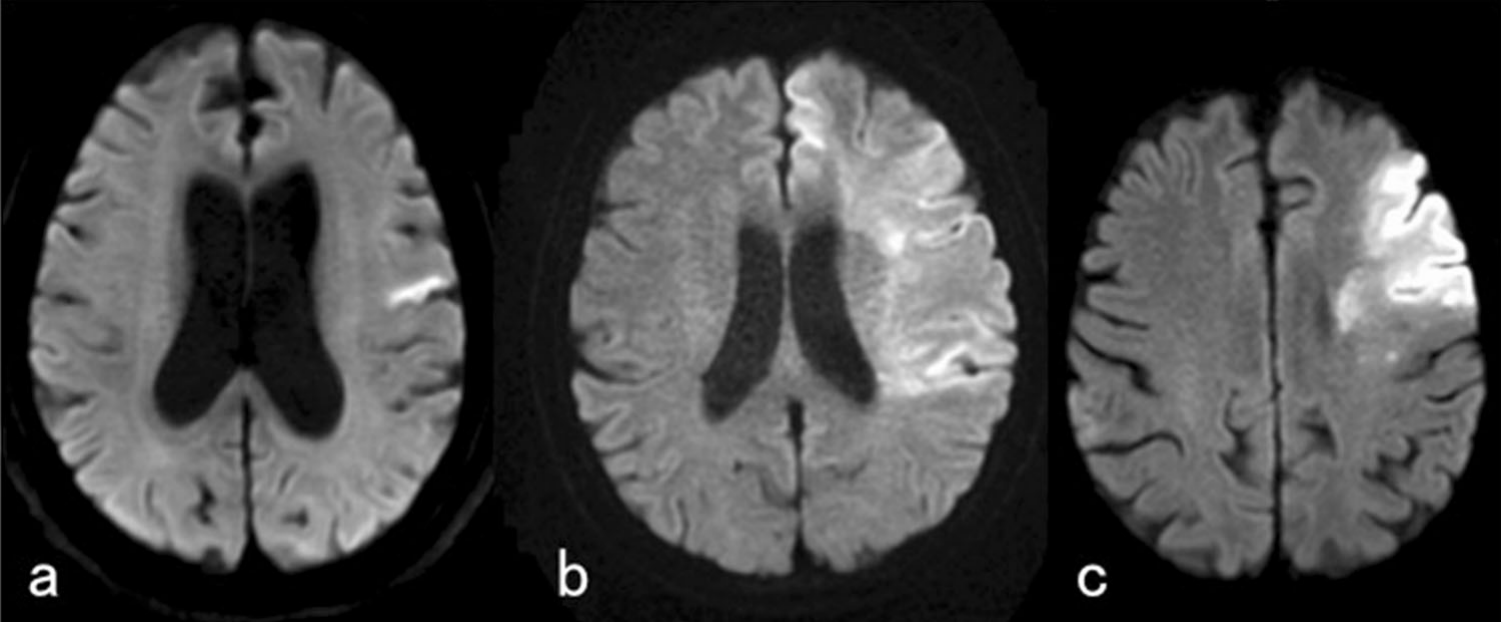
Diffusion-weighted magnetic resonance imaging (DWI) of three representative cases. a Case 1 demonstrated a left hemisphere lesion including the entire frontal lobe, primary sensory cortex, angular gyrus, and insula. b Case 2 suffered an extended left hemisphere lesion including the entire frontal lobe, primary sensory cortex, angular gyrus, and insula. c Case 3 had a relatively broad infarction including orbitofrontal cortex, inferior frontal gyrus, and insula of the left cerebral hemisphere; however, large parts of the middle frontal gyrus and primary motor cortex were spared
